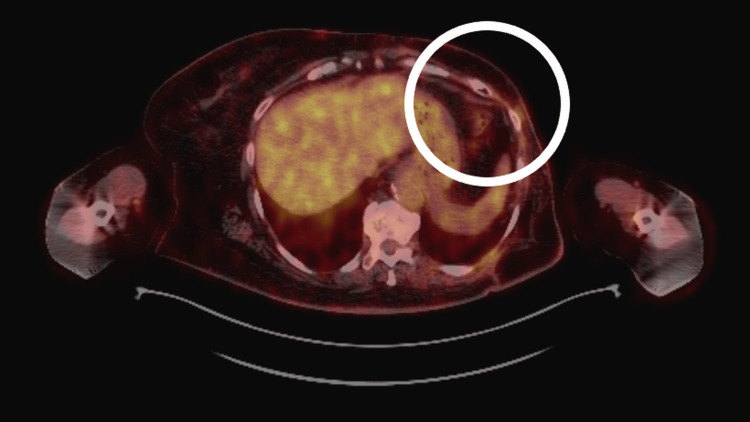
Follow-up PET/CT imaging (December 2025). Axial PET/CT image demonstrating persistent focal fluorodeoxyglucose uptake in the left anterior chest wall (circled), consistent with ongoing metabolically active localized disease. No evidence of lymph node or distant organ involvement is identified.
